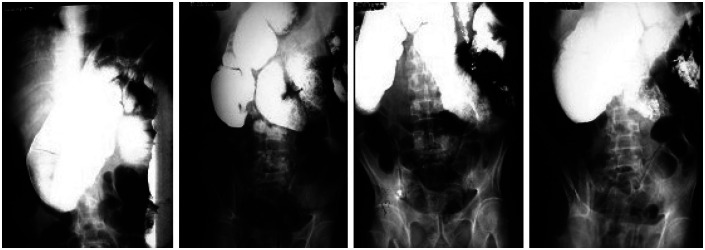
Radiographic examination suggesting dilatation without stenosis in a case of chronic idiopathic intestinal pseudo-obstruction.
